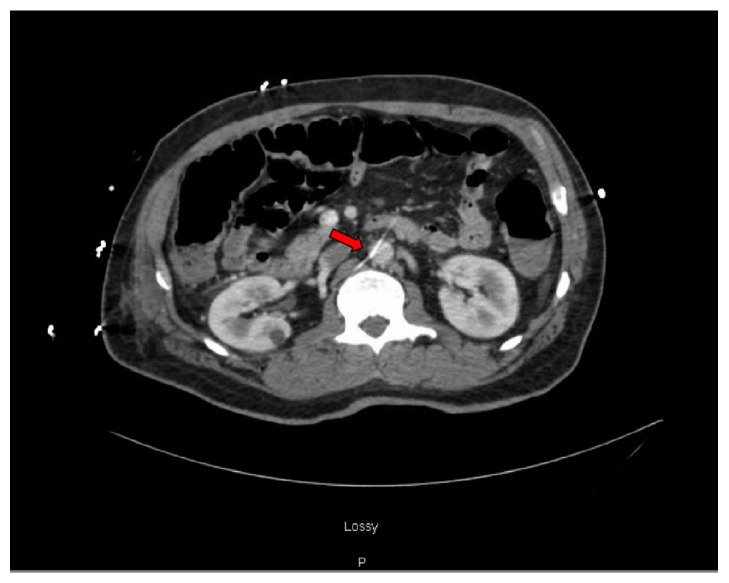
CT abdomen/pelvis findings showing the inferior metallic marker of the intra-aortic balloon pump below the origin of the superior mesenteric artery (arrow).
